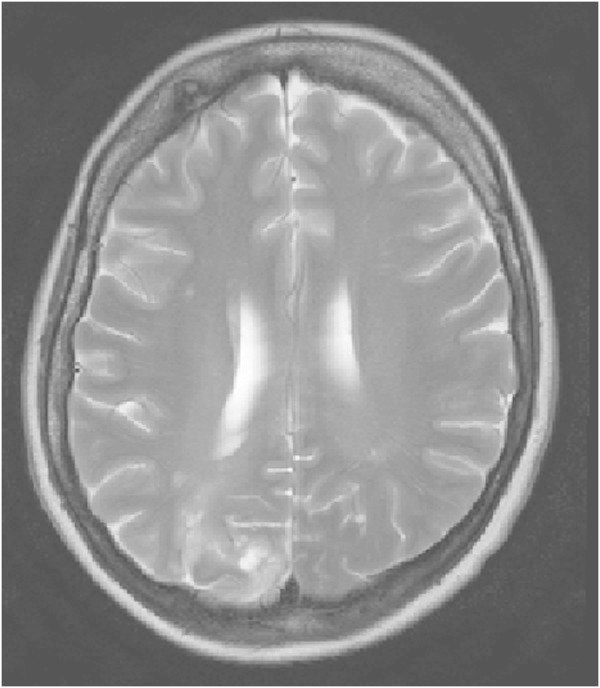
MRI after 5 months, T2-weighted image.
